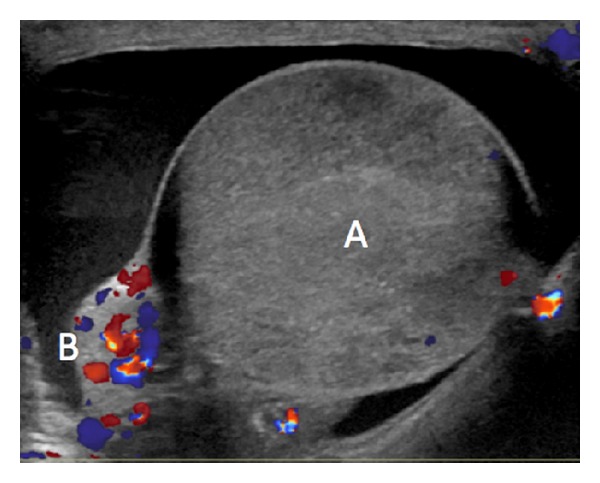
Right scrotal ultrasound with color Doppler interrogation on day 7 of hospitalization showing A: right markedly heterogeneous testis with blood flow essentially absent; B: prominent, hyperemic right epididymis.
